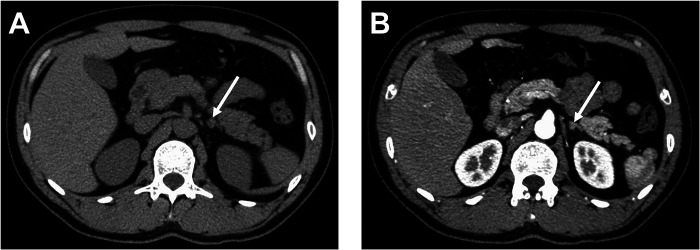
CT of patient before operation. (A) Plain CT. (B) Contrast-enhanced CT scan.
